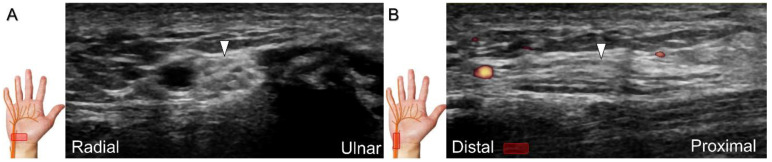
Sonographic images depict a fibrolipomatous hamartoma (arrowheads) in the short- (A) and long-axis (B) views.
